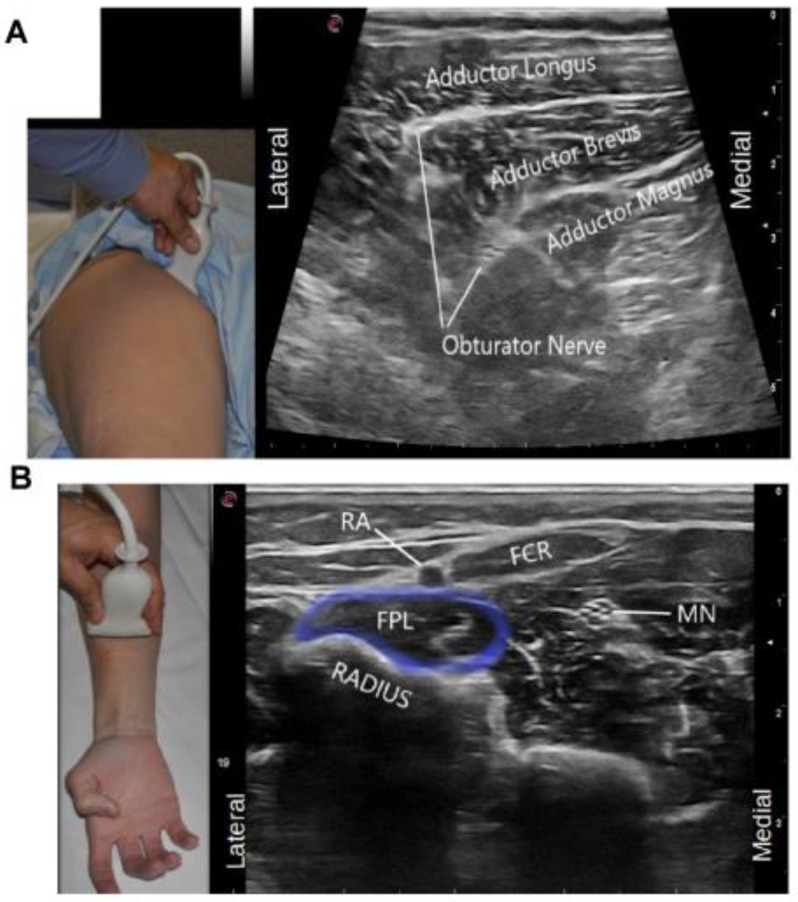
Visual Ergonomics. (A) Anterior and posterior branches of the obturator nerve can be used to delineate the fascial planes of the adductor longus, brevis and magnus (B) Visualisation of the median nerve can be used as landmark to identify the Flexor Pollicis Longus. Identification of these nerve to muscle relationships can also be key if nerve blocks are to be performed for diagnostic injection to determine contracture versus spasticity (FCR: Flexor Carpi Radialis, RA: Radial artery, MN: Median Nerve, FPL: Flexor Pollicis Longus). (Images acquired on Esaote MyLab 7 ultrasound machine, Esaote ultrasound machine with 13–3 Hz linear array transducer, (Esaote; location: Fishers, IN, USA)).
